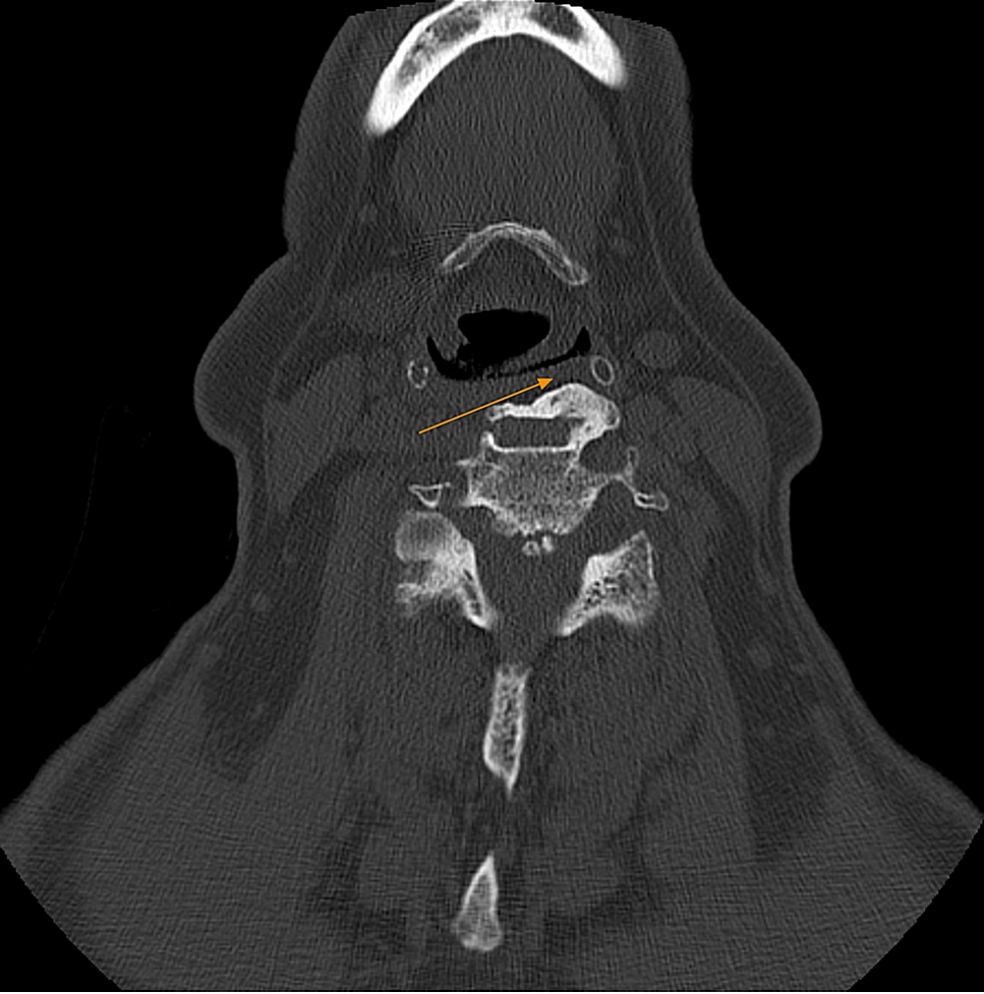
Transverse computed tomography demonstrating a bony bridge between adjacent osteophytes, causing a left impression on the airway. The arrow pointing to the osteophytes at the C2-C3 vertebral level.
